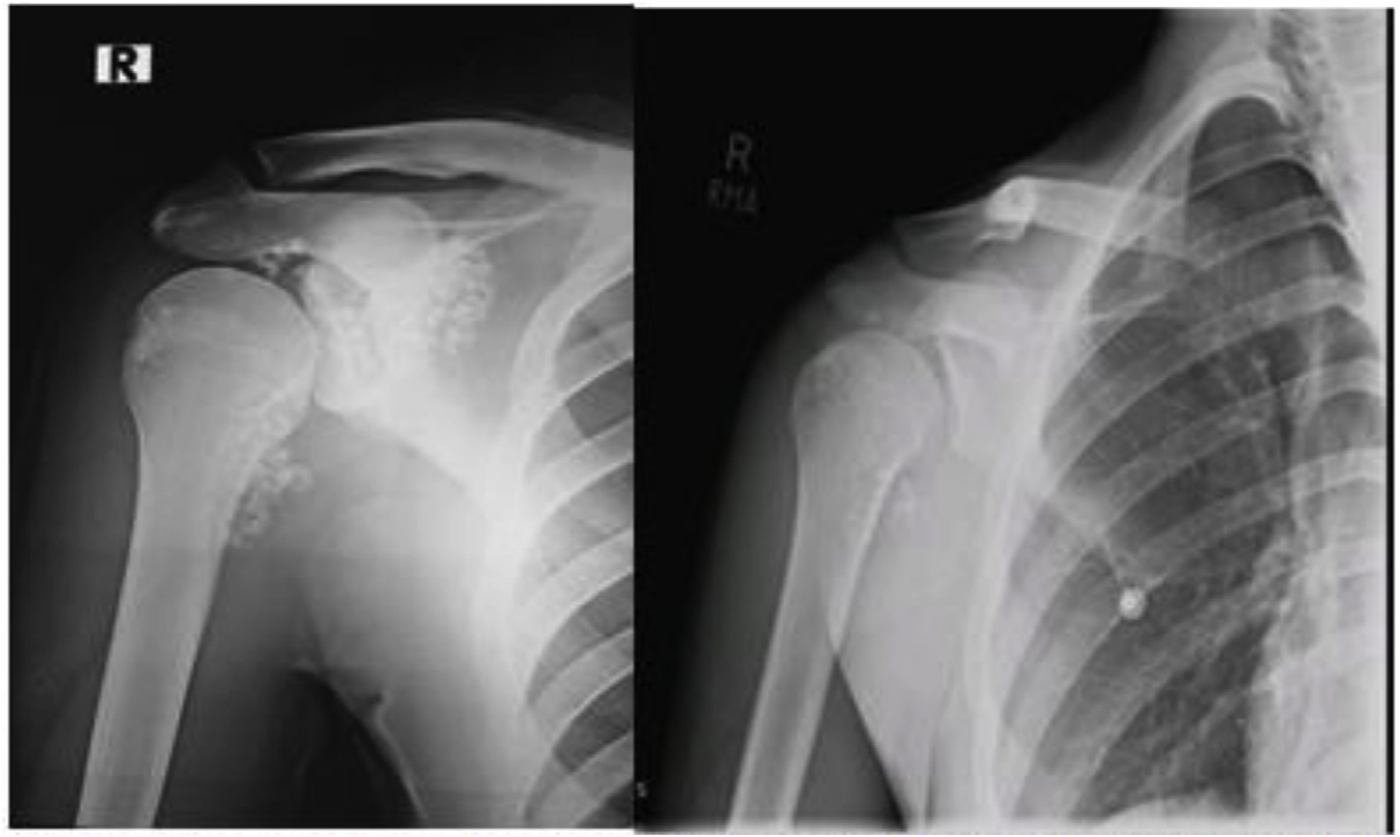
On left side pre operative x-ray showing the extensive loose bodies in shoulder joint on right side postoperative x-ray after removal of the loose bodies.
